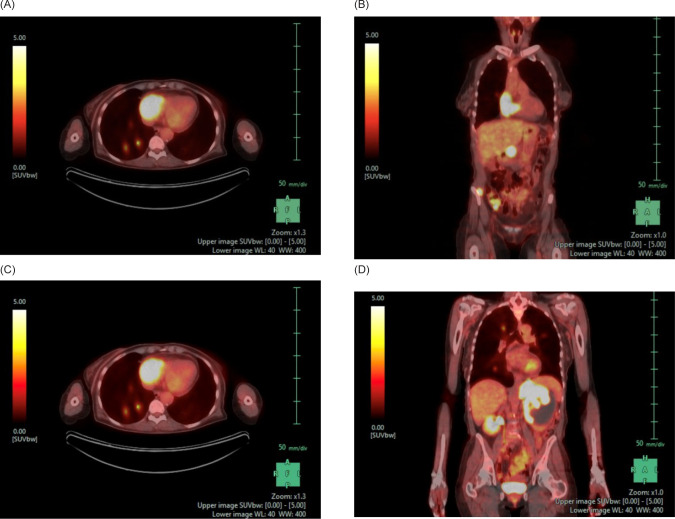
PET-CT of the chest, abdomen, and pelvis. (A) Axial and (B) coronal views demonstrating a FDG uptake of the right atrium suggestive of a cardiac metastasis. There are also FDG avid pulmonary nodules. (C) Axial and (D) coronal views demonstrating a heterogeneously attenuating 9.6 × 8.7 cm left renal mass and accompanying 5.9 × 4.3 FDG avid adrenal mass. There is also a 2.6 × 1.2 cm FDG avid right adrenal metastasis and multiple FDG avid abdominopelvic nodules.
